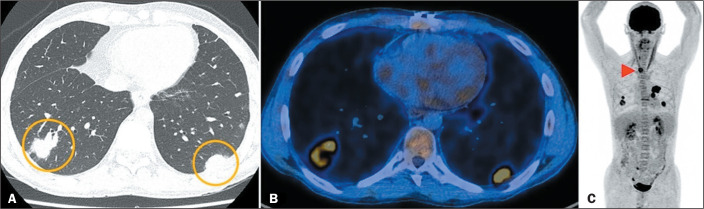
PTLD. A: CT scan showing sparse rounded parenchymal opacities
